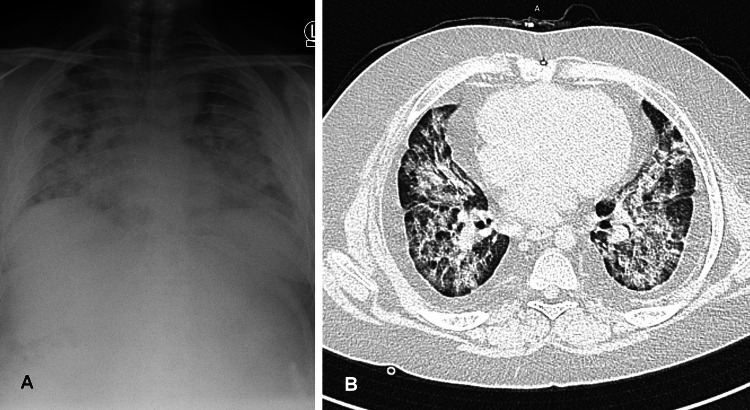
Radiological findings on chest X-ray and CT scan of the second patient (A) Chest X-ray, PA view: massive bilateral inflammatory changes. (B) Chest CT, transverse plane: lungs with reduced volume, extensive bilateral parenchymal and interstitial densities, multifocal ground-glass opacity. Changes are more intense in the right lung, involving approximately 60% of the lung parenchyma. CT: computerized tomography; PA: posteroanterior.
